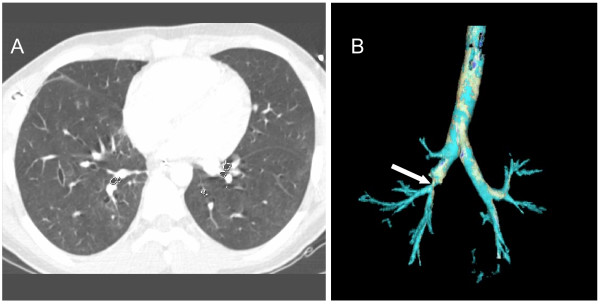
Computed tomography scan performed 2 weeks following surgery. (a) Complete recovery of the pulmonary contusion (axial slice at the level of the lower lobes). (b) The three-dimensional reconstruction of the tracheobronchial tree, however, demonstrates a bronchial stenosis (white arrow) at the site of surgical repair.
